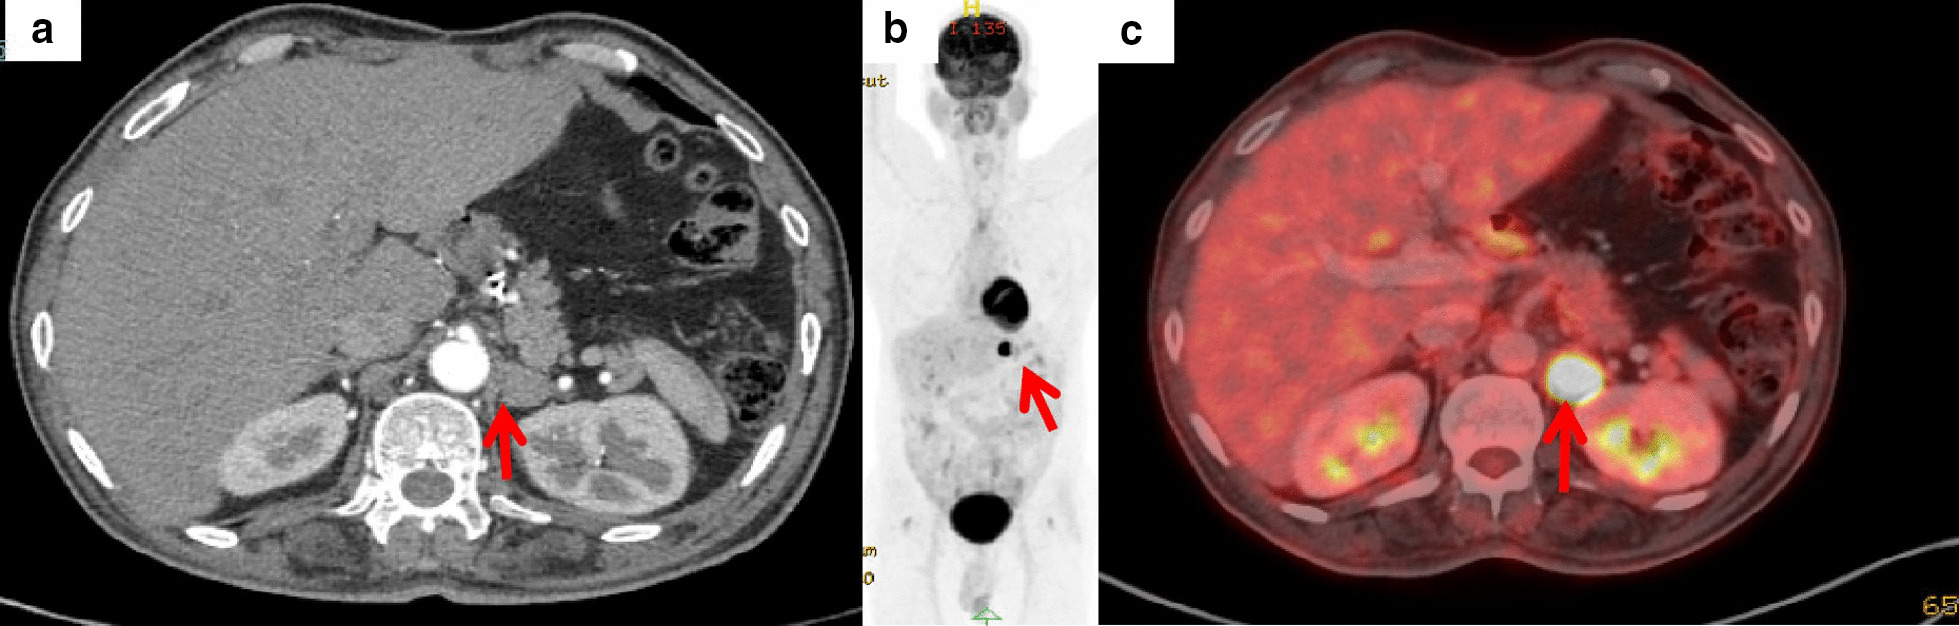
a Axial image of the CT scan showing the left adrenal lesion (red arrow); b maximum intensity projection image of PET-CT; and c axial image showing uptake in the left adrenal lesion (red arrow)
